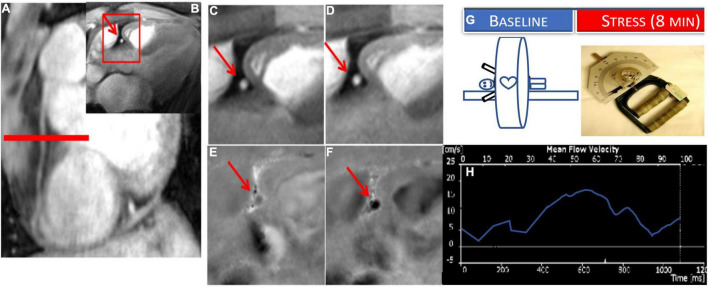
Example of coronary endothelial function (CEF) testing using non-contrast MRI with isometric handgrip exercise (IHE). Scout MRI (A) and cross-sectional cine (B–D) and phase-contrast images (E,F) in a healthy subject showing RCA in cross-section (red arrow). In the expanded inset sections, coronary area increases from rest (C) to IHE (D) and velocity and flow increase from rest (E) to stress (F) (note that increased darkness represents increased signal and thus velocity down through the imaging plane). (G) Stress MRI protocol for CEF measures for endpoints: change in coronary cross sectional area and blood flow velocity (%) from baseline to stress (continuous IHE for 5–8 min). (H) Example coronary flow velocity curve of RCA.
